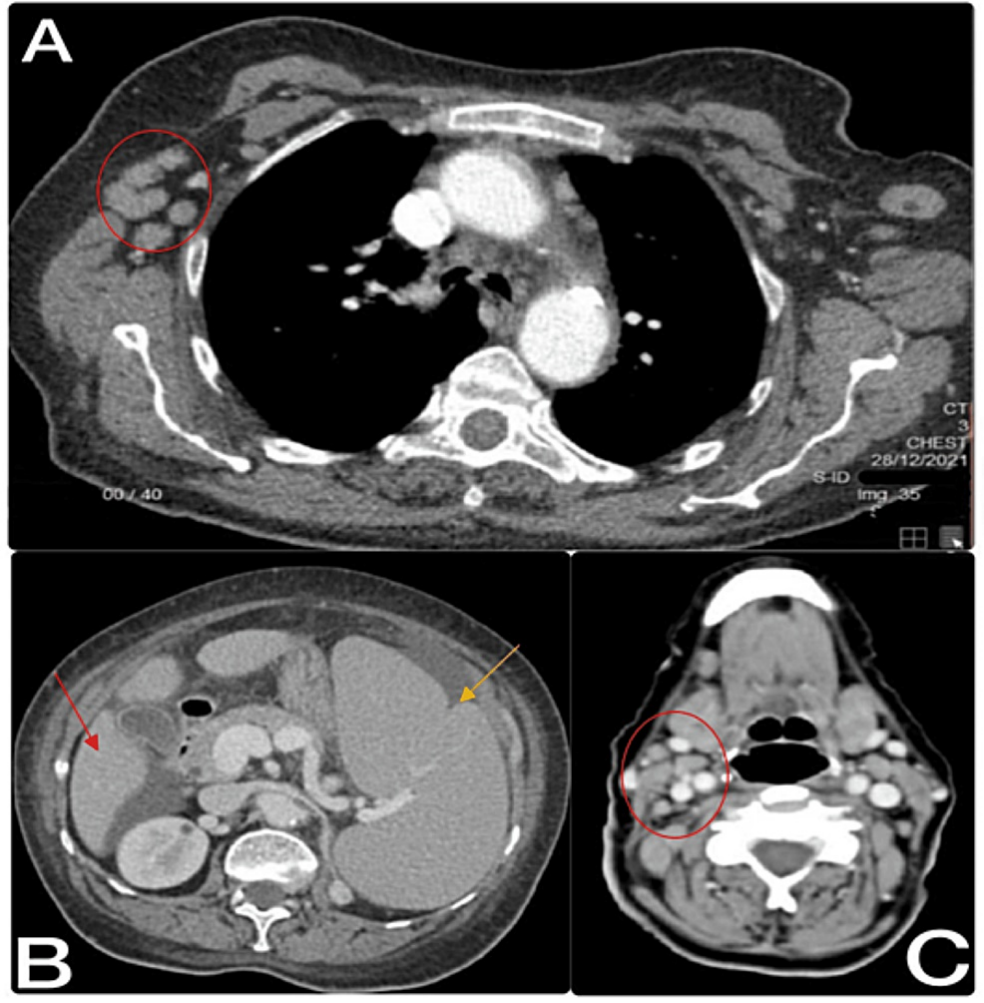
Computed tomography (CT). (A) CT chest showing multiple enlarged axillary lymph nodes (red circle). (B) CT abdomen showing hepatosplenomegaly with minimal ascites (red arrow spleen, yellow arrow liver). (C) CT neck showing multiple prominent upper neck lymph nodes (red circle cervical lymph node).
